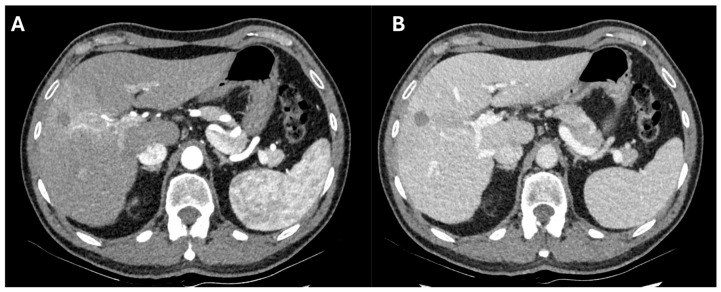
Computed tomography arterial (A) and portal (B) phase of a case of successful downstaging by a combination of loco-regional therapies and immunotherapy. After a microwave ablation of a Sg8 HCC, the patient developed a neoplastic thrombosis of Sg8 Glissonean pedicle. He was treated with trans-arterial radio-embolization followed by 20 cycles of Atezolizumab–Bevacizumab. After disappearance of contrast-enhancing tissue at the Sg8 pedicle and normalization of alpha-fetoprotein (from 42.9 to 8.1 ng/mL), the patient was waitlisted for liver transplantation 1 month after the last administration of immunotherapy. He was transplanted 10 days after and had an uneventful postoperative course, with no sign of acute rejection. At 5-month follow-up, he had normal liver function and no evidence of recurrence.
